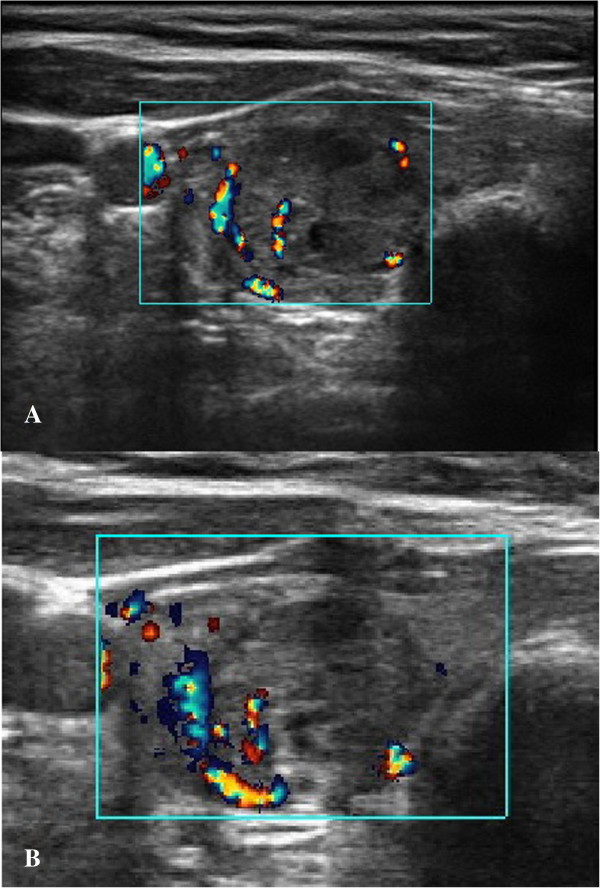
Doppler US image of exemplary thyroidal nodule pre- (A) and 1 day postintervention (B).
